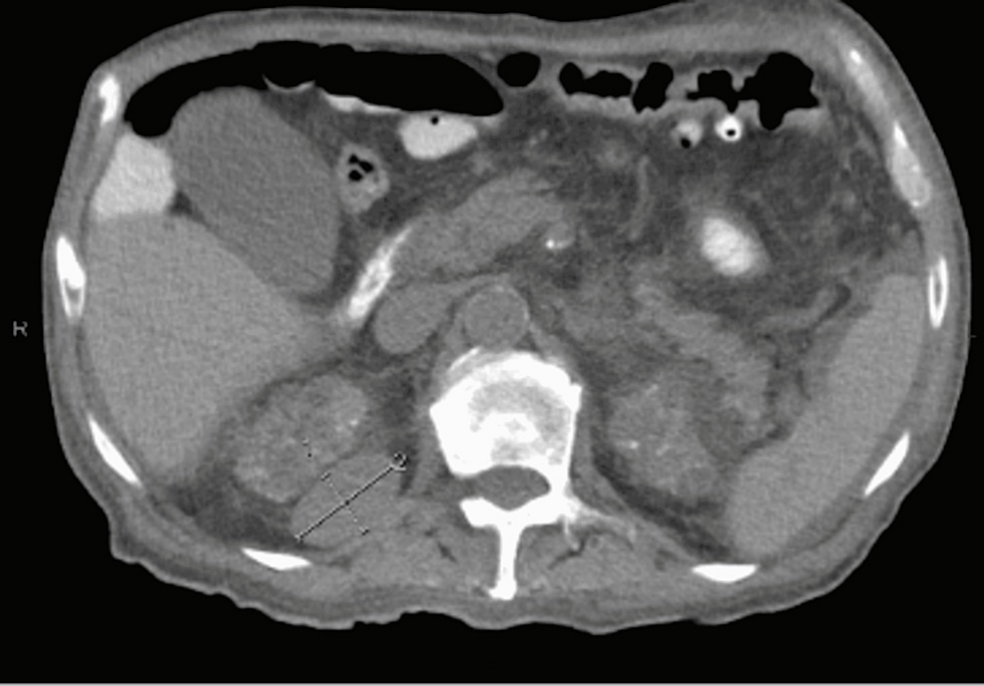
CT of the abdomen demonstrating a 4.1 cm × 2.5 cm left retroperitoneal nodule, suspicious for a metastatic lesion. CT: computed tomography
